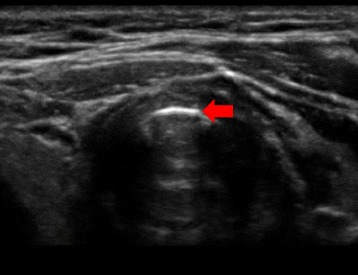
Ultrasound-guided identification of the cricothyroid membrane This is a cross-sectional view of the cervical ultrasound image. The red arrow shows the cricothyroid membrane. The cricothyroid membrane could not be identified by palpation, but was identified on ultrasound
